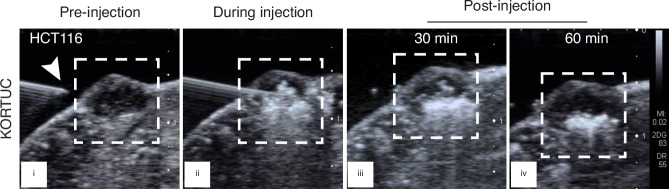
Ultrasound guided intratumoural administration of KORTUC in HCT116 tumour xenografts demonstrating sustained presence of O_2_ microbubbles. US images of an HCT116 tumour (i) pre-injection, (ii) during injection, and (iii) 30 min and (iv) 60 min post-injection of KORTUC. Oxygen microbubbles were seen as a persistent white haze in images (ii)–(iv). The white arrow indicates the needle entry point and the dashed box delineates the tumour region. Reverberation apparent below the needle is an US imaging artefact [36, 37].
